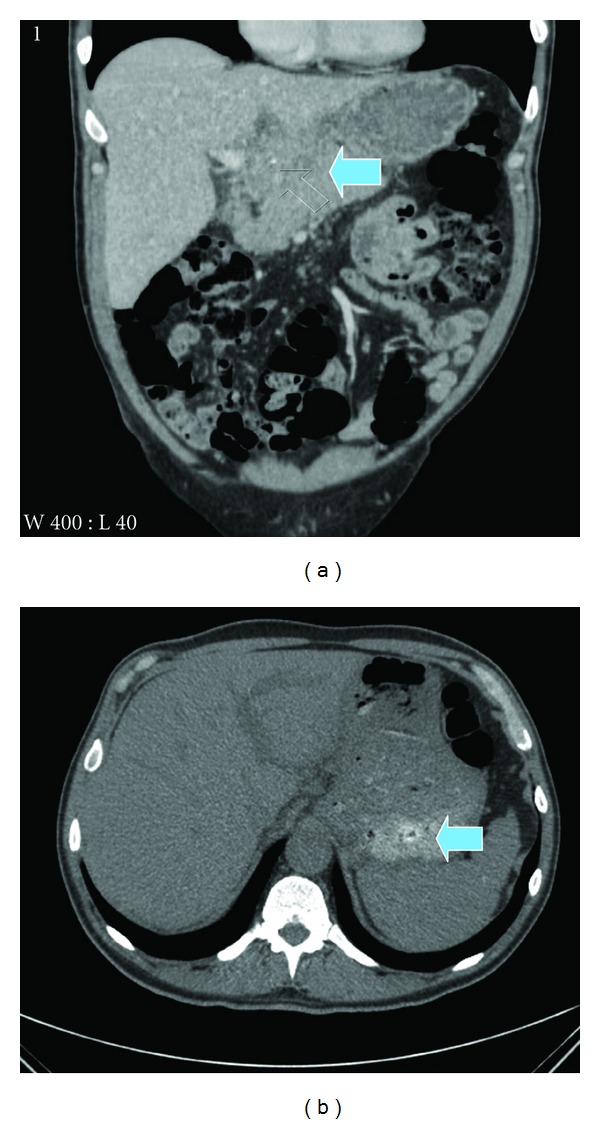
CT scan revealing a complex soft tissue mass in relation to the pancreas with a calcified focus.
